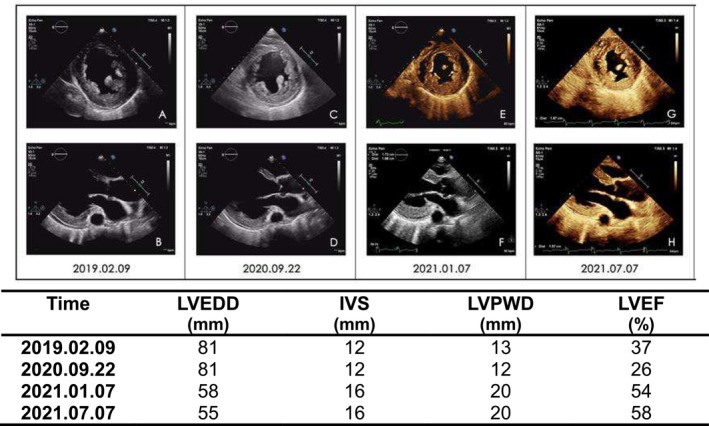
Echocardiograms from left parasternal short‐axis view (A, C, E, G) and apical four‐chamber view (B, D, F, H) showing significant reversal of cardiac remodeling. LVEDD: Left ventricular end‐diastolic diameter; IVS: Interventricular septum; LVPWD: Left ventricular posterior wall thickness; LVEF: Left ventricular ejection fraction.
